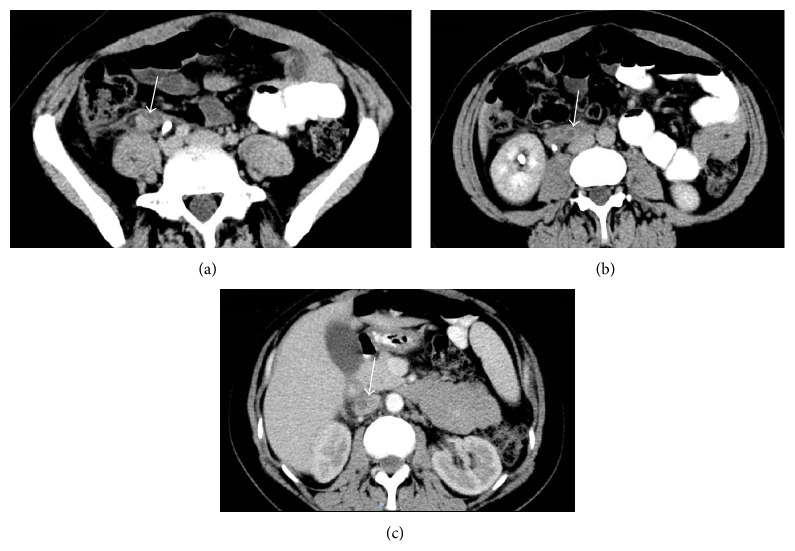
Axial contrast-enhanced CT images demonstrate enlargement of right gonadal (ovarian) vein with central hypodensity and enhancement of vessel wall (a-b) with cephalad extension to inferior vena cava (c). Inflammatory changes in adjacent retroperitoneal fat can also be seen.
